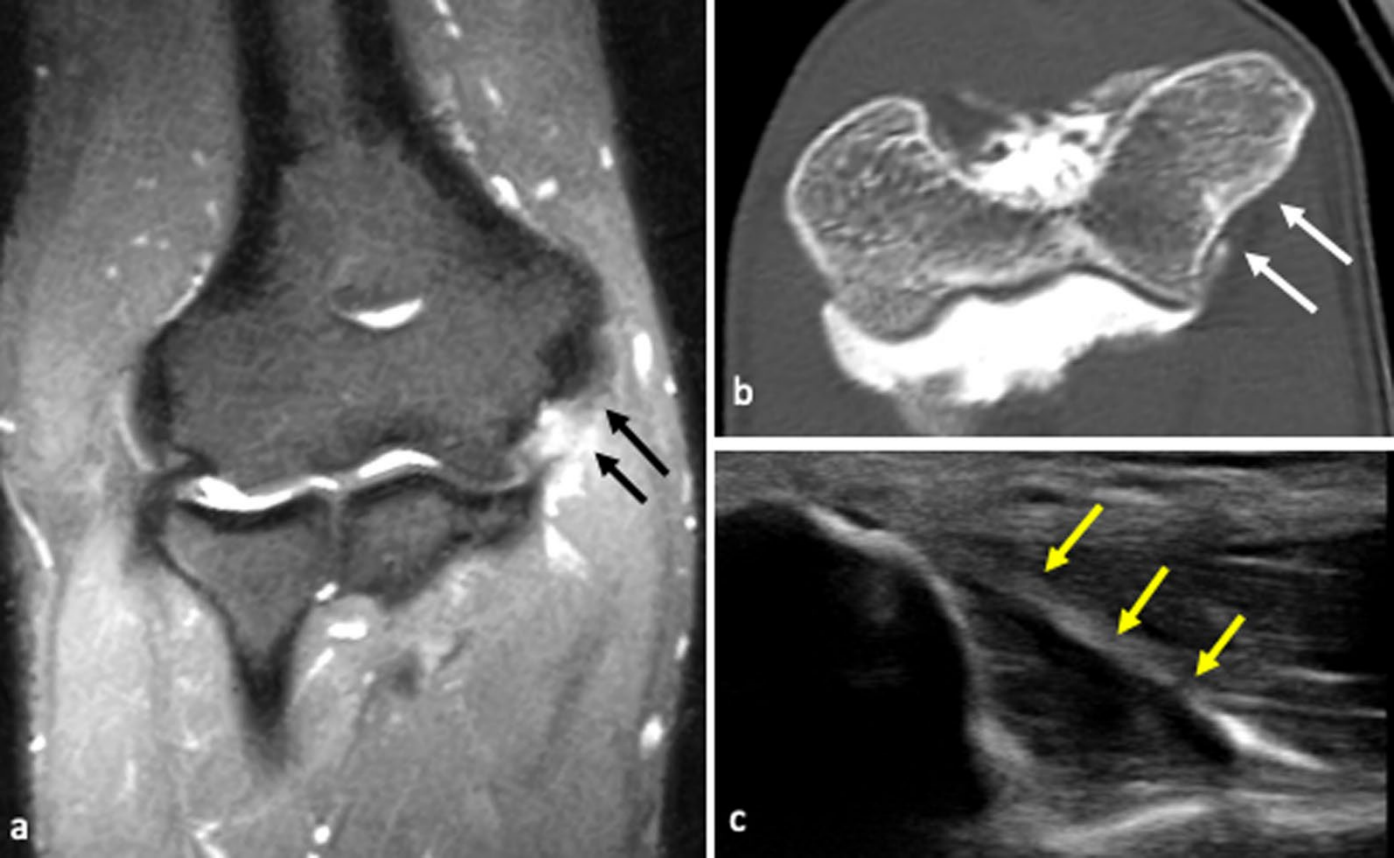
Professional tennis player with chronic medial elbow pain, exacerbated during serve, not responsive to medical and physical treatments. MRI coronal fat-suppressed T2-weighted images (a) show a high-intensity signal at the anterior bundle (A-MCL), suggestive of partial tear (black arrows). CT-arthrography reformatted images along the major axis of the anterior bundle (b) demonstrate a thickened but continuous ligament (white arrows). US scan prior to contrast agent injection (c) confirms diagnosis by showing a thickened, hypoechoic ligament with irregular margins, although regularly inserted (yellow arrows)
